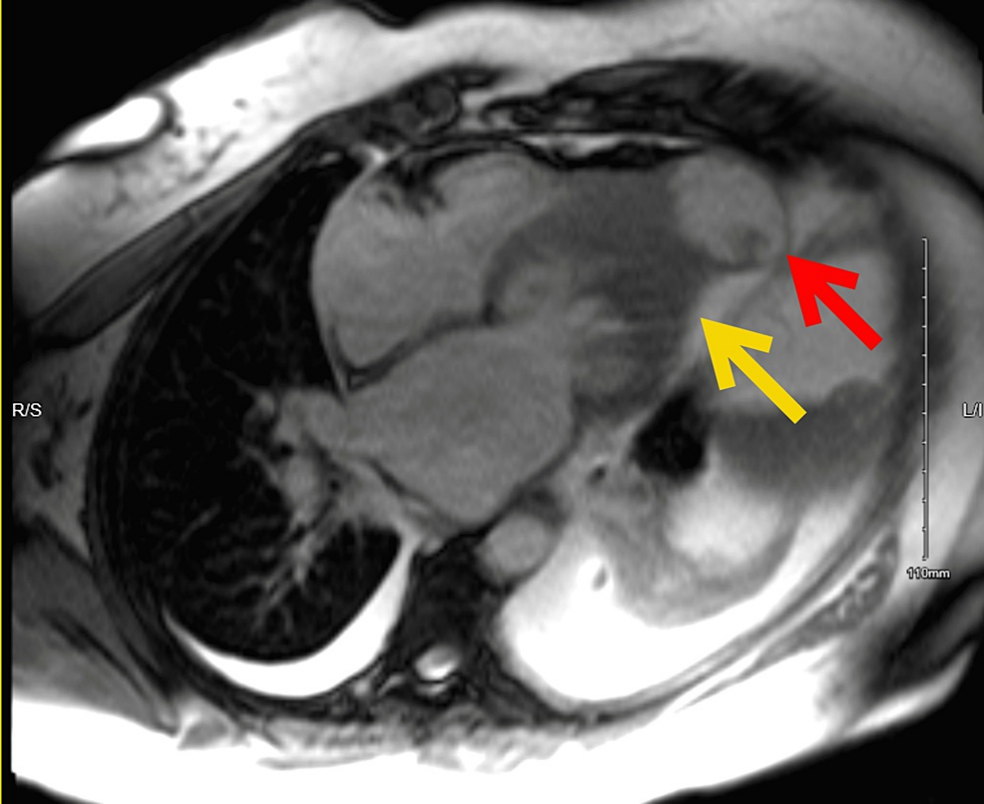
Cardiac MRI axial view showing apical hypertrophy indicated with a yellow arrow and an apical aneurysm with a 21 x 14 mm thrombus within it indicated with a red arrow
